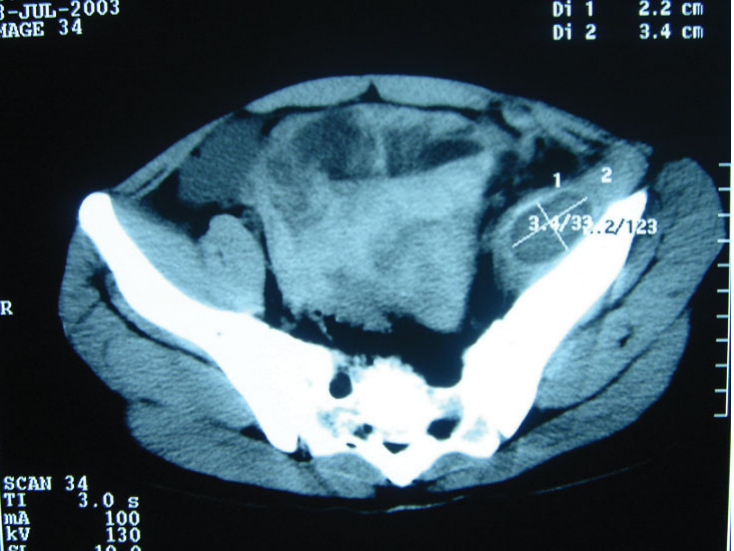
Plain CT scan. Diffuse swelling is seen in the left iliopsoas muscle and there is a ring-like hypodense local swelling with a high-density rim noted in the left iliacus muscle.
